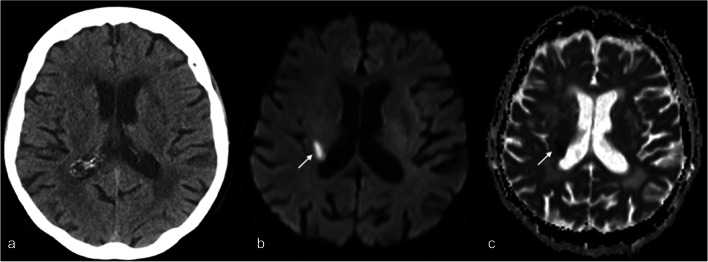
Representative CT-occult minor stroke in a patient presenting with transient left-sided mild hemiparesis. Non-contrast CT (a) did not show any signs of ischemic stroke. Diffusion-weighted imaging (b, b1000) and the corresponding apparent diffusion coefficient map (ADC map, c) from the short MRI protocol revealed an ischemic lesion in the posterior limb of the right internal capsule (arrow)
